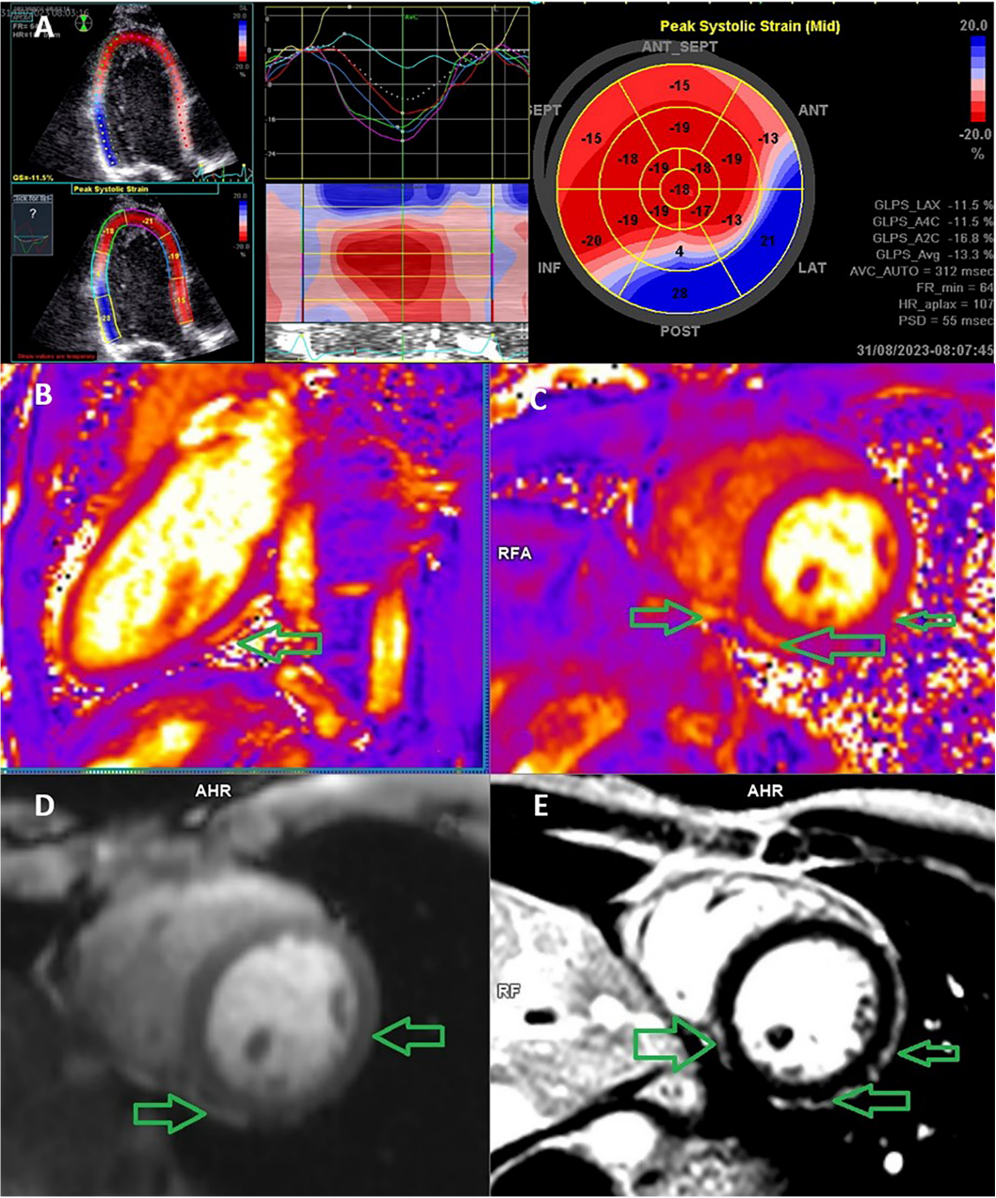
Echocardiography speckle-tracking strain analysis and cardiac MRI in a 17-year-old man with acute perimyocarditis. (A) Speckle-tracking assessment of segmental and global strain on an apical long-axis echocardiography view with a bulls-eye plot of regional longitudinal strain values, which demonstrates impaired strain at the basal inferior and inferolateral wall. The blue segment is dyskinetic, meaning that the wall segment is bulging when it should be contracting. If the left ventricle walls are dyskinetic (i.e., bulging out), the GLS value is positive. (B) Cardiac MRI image demonstrates a T2 relaxometry native dual-chamber scan; the arrow indicates the area of the perimyocardial edema. (C) T2 relaxometry native short axis scan; the arrow indicates the area of the perimyocardial edema. (D) T1 DCE (dynamic contrast enhancement)—early enhancement of the inflammation in the peri-myocardial position of the chamber wall showing an enhancement of the near pericardial layer typical of myocarditis. (E) T1 PSIR postcontrast 15 min, short-axis scan, the arrow indicates the area of peri-myocardial scarring. The left ventricular ejection fraction (global function) was reduced. MRI: magnetic resonance imaging.
